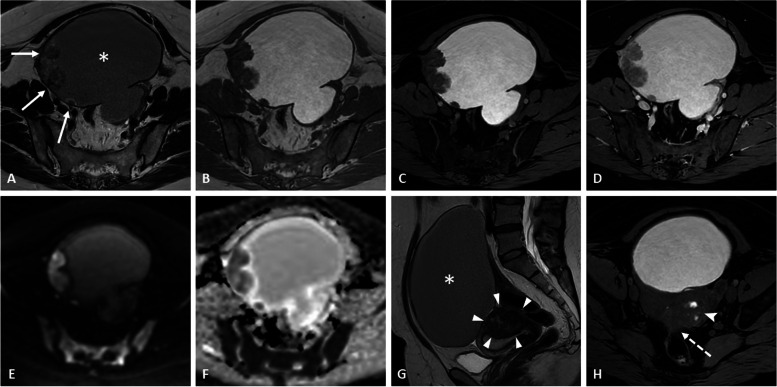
Endometrioid adenocarcinoma within a right ovarian endometrioma in a 43-year-old woman without any medical history. Axial T2-weighted image (a) shows a large ovarian cystic mass with chronic bleeding (asterisk) displaying a “T2-shading” appearance and peripheral wall nodules (arrows). The axial T1-weighted (b) and fat-suppressed T1-weighted (c) images confirm the hemorrhagic cystic content. Enhancement of the nodules is seen on fat-suppressed T1-weighted following gadolinium chelates injection (d). The axial diffusion image (e) shows the high signal intensity regarding neoplastic nodules, with corresponding low intensity on the ADC map (f). Sagittal T2-weighted image (g) shows features of pelvic endometriosis with uterine adenomyoma (arrowheads) and several hemorrhagic foci (arrowhead) with anterior rectal wall involvement (dotted arrow) on axial fat-suppressed T1-weighted image (h)
